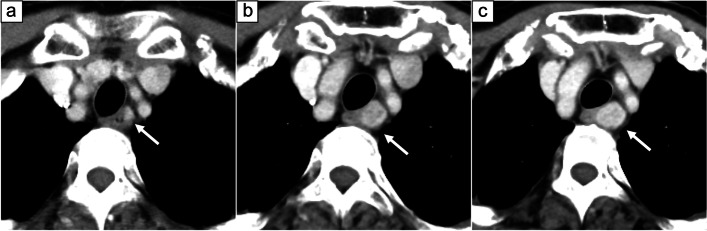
Contrast-enhanced CT findings. An internally heterogeneous, nodular lesion with contrast effect was observed on the left side of the cervical esophagus (white arrow). The maximum diameter of the lesion was a 7 mm in July 2019, b 14 mm in December 2020, and c 17 mm in May 2021, demonstrating rapid growth over about 2 years
